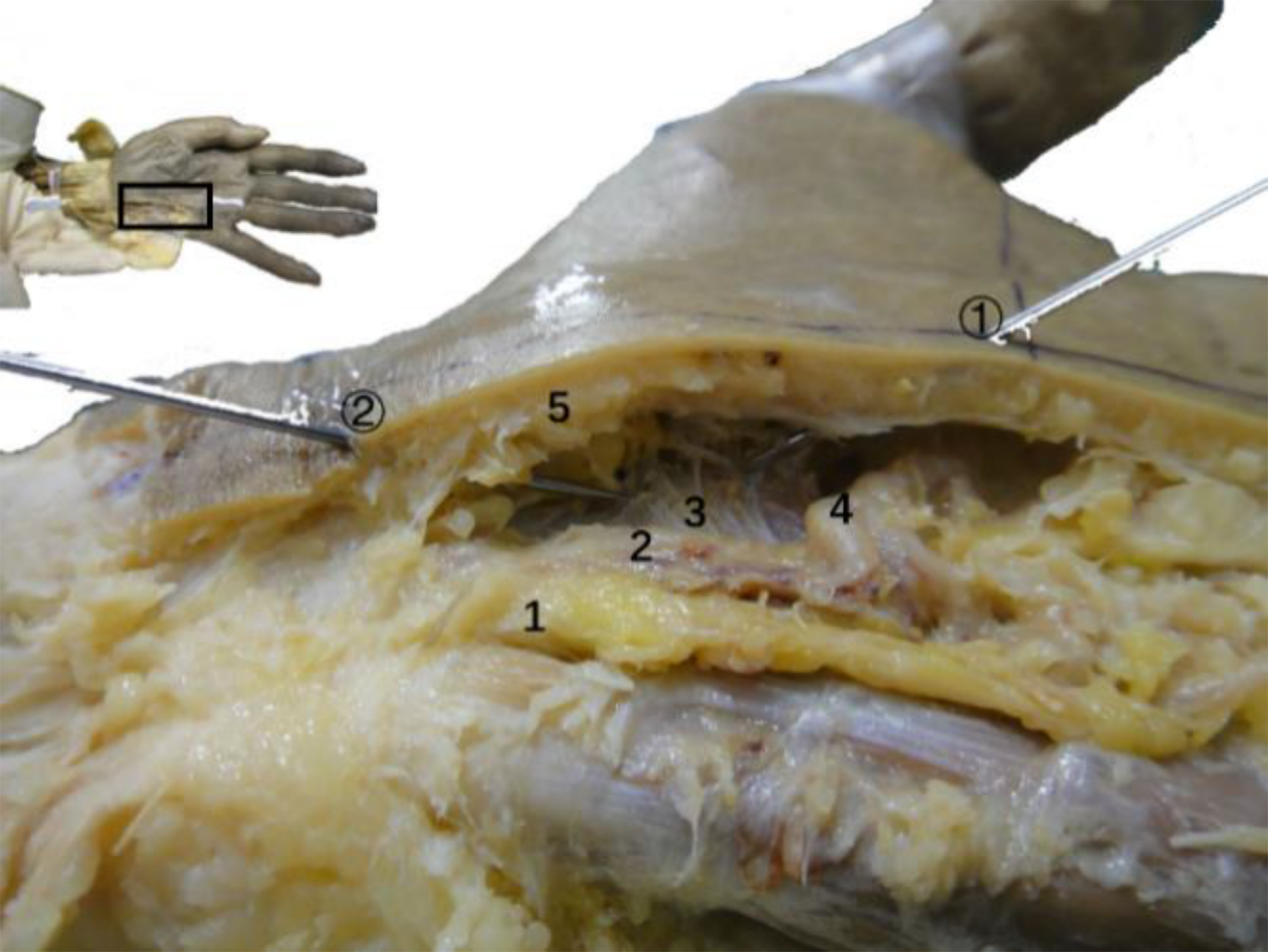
The longitudinal section of the needle-knife release technique guided by ultrasound. 1, ulnar nerve; 2, ulnar artery; 3, transverse carpal ligament; 4, superficial palmar arch; 5, palmar aponeurosis (section); ①, needle entry point 1; ②, needle entry point 2.
